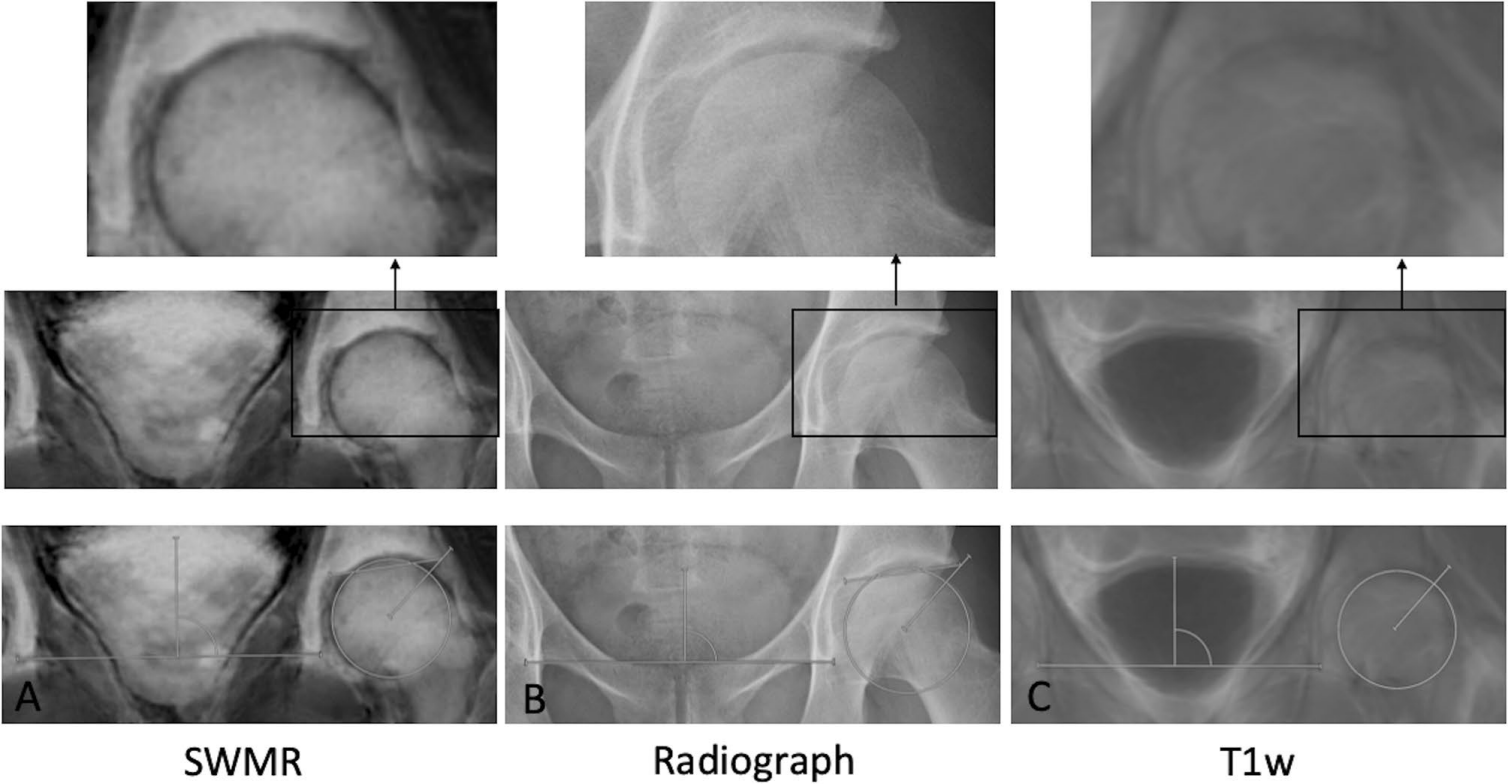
Example for angle measurements. Radiograph in anteroposterior view (B) and coronal maximum intensity projections of susceptibility-weighted MRI magnitude images (SWMR; A) and T1weighted MRI (T1w; C) with magnifications and angle measurements. Tönnis angle could not be measured on T1w because the acetabular sourcil could not be identified reliably.
